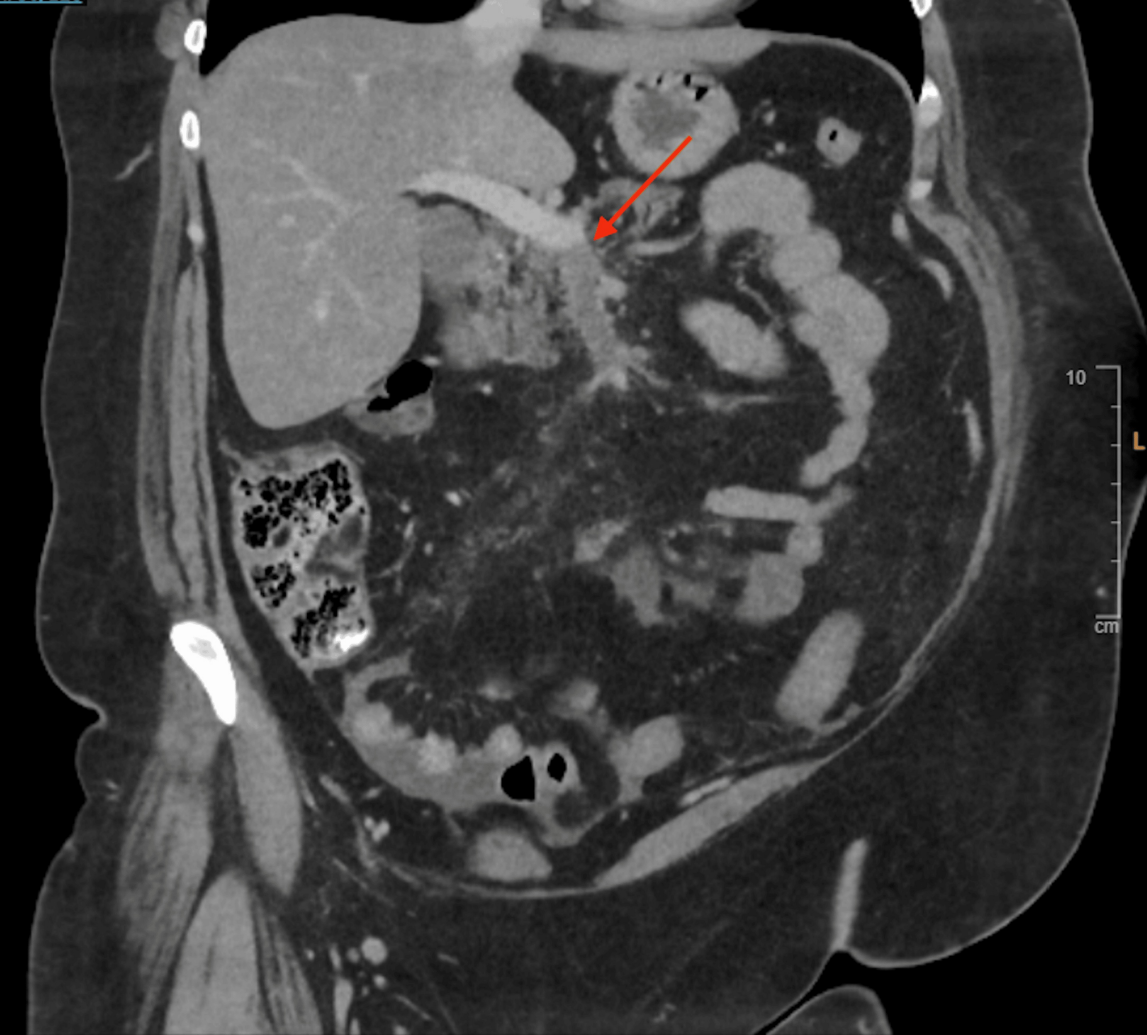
Coronal contrast-enhanced CT scan showing acute superior mesenteric vein thrombosis (red arrow).
